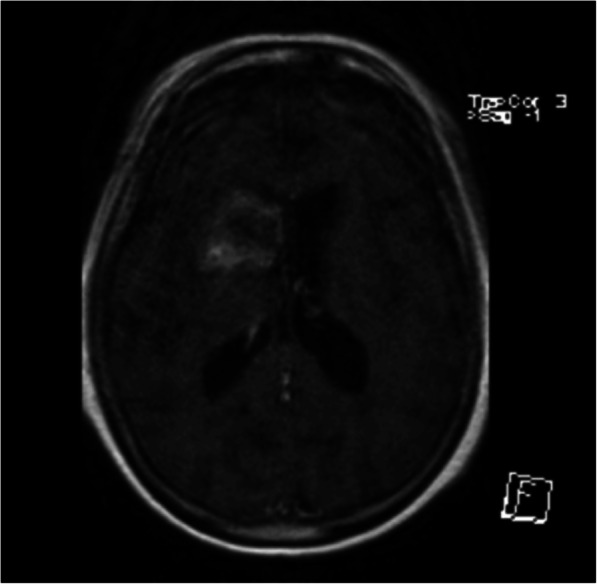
Axial T1-weighted MRI demonstrating right sided MCA territory infarction
